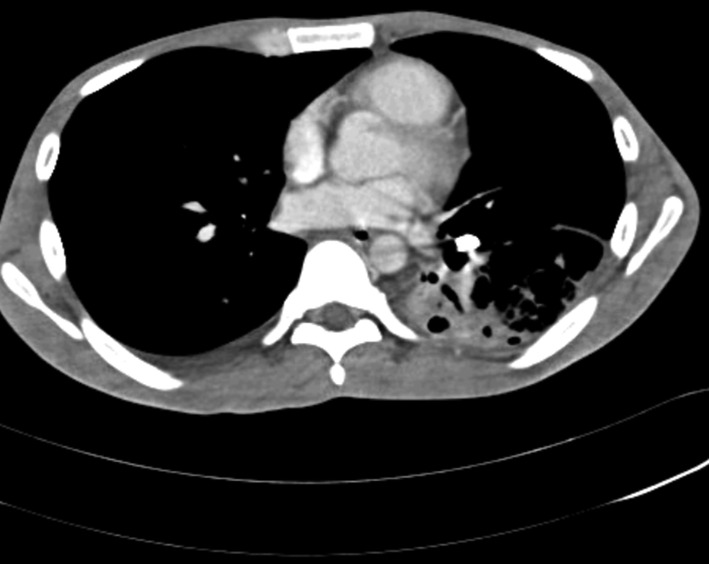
Contrast‐enhanced computed tomography showing a hyperdense foreign body (screw) lodged in the left lower lobe bronchus, with left lower lobe cavitating consolidation, centrilobular and tree‐in‐bud nodules noted in bilateral lung fields
